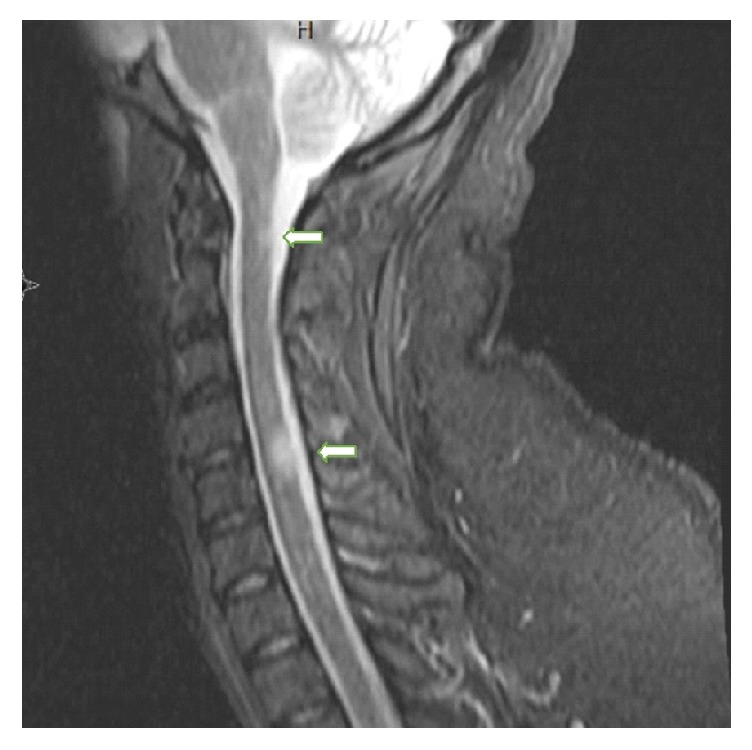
Sagittal T2-weighted images of the cervical spine showing abnormal signal cord at C2 and C4-5 level (arrows).
